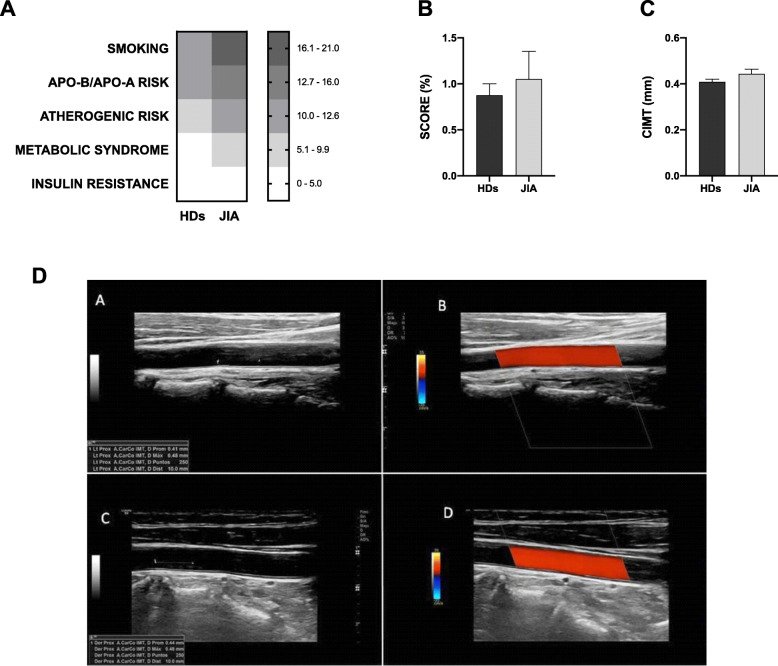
CVD risk factors and subclinical atherosclerosis in JIA patients in remission. a Heatmap of CVD risk factors between JIA patients and HDs. Data expressed in percentage (%). b SCORE CVD risk in JIA patients compared to HDs. c CIMT in JIA patients compared to HDs. d a: HD greyscale cIMT; b: HDs Power Doppler cIMT; c: JIA greyscale cIMT; d: JIA Power Doppler cIMT. CVD, cardiovascular disease; JIA, juvenile idiopathic arthritis; HDs, Healthy donors
